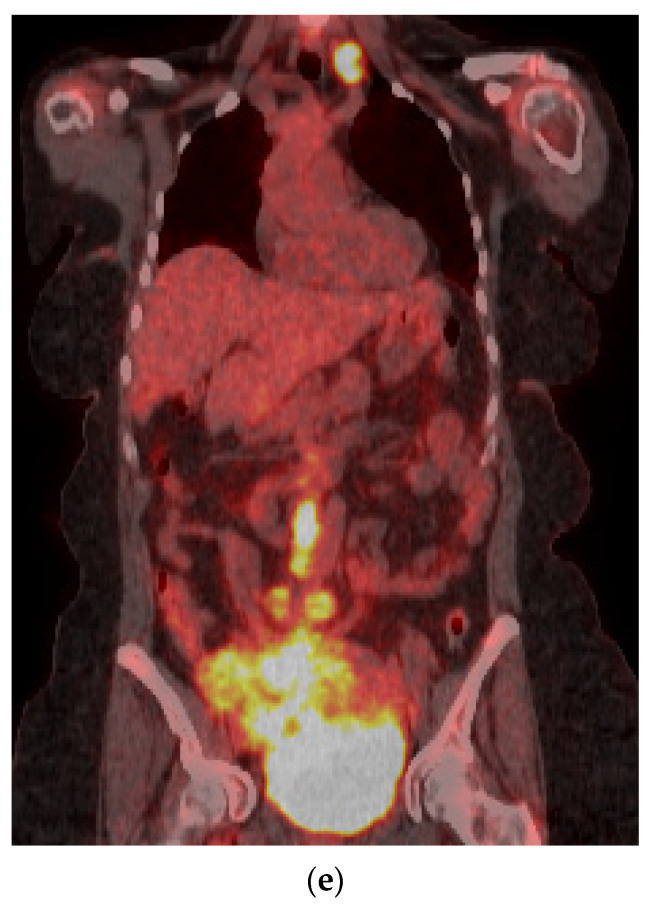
Marked progression of locally advanced bladder cancer and metastatic disease. (a) Axial CT image demonstrates a small lesion in the anterior bladder which went untreated for 16 months. (b) Follow up axial CT image showing significant progression of the mass which now extends beyond the bladder to involve the uterus, right adnexa, and right pelvic side wall. (c) Axial PET/CT image redemonstrating the locally advanced mass with bilateral pelvic lymphadenopathy. (d) Axial PET/CT image shows metastatic retroperitoneal lymphadenopathy. (e) Coronal PET/CT image also showing metastatic left supraclavicular lymphadenopathy. Biopsy of the left supraclavicular node showed poorly differentiated carcinoma compatible with bladder primary.
